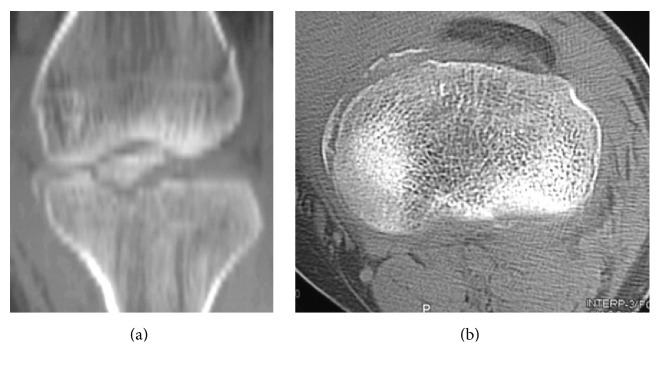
Computed tomography: (a) coronal image showing tibial eminence and reverse Segond fractures and (b) axial image showing reverse Segond and anteromedial rim fractures.
